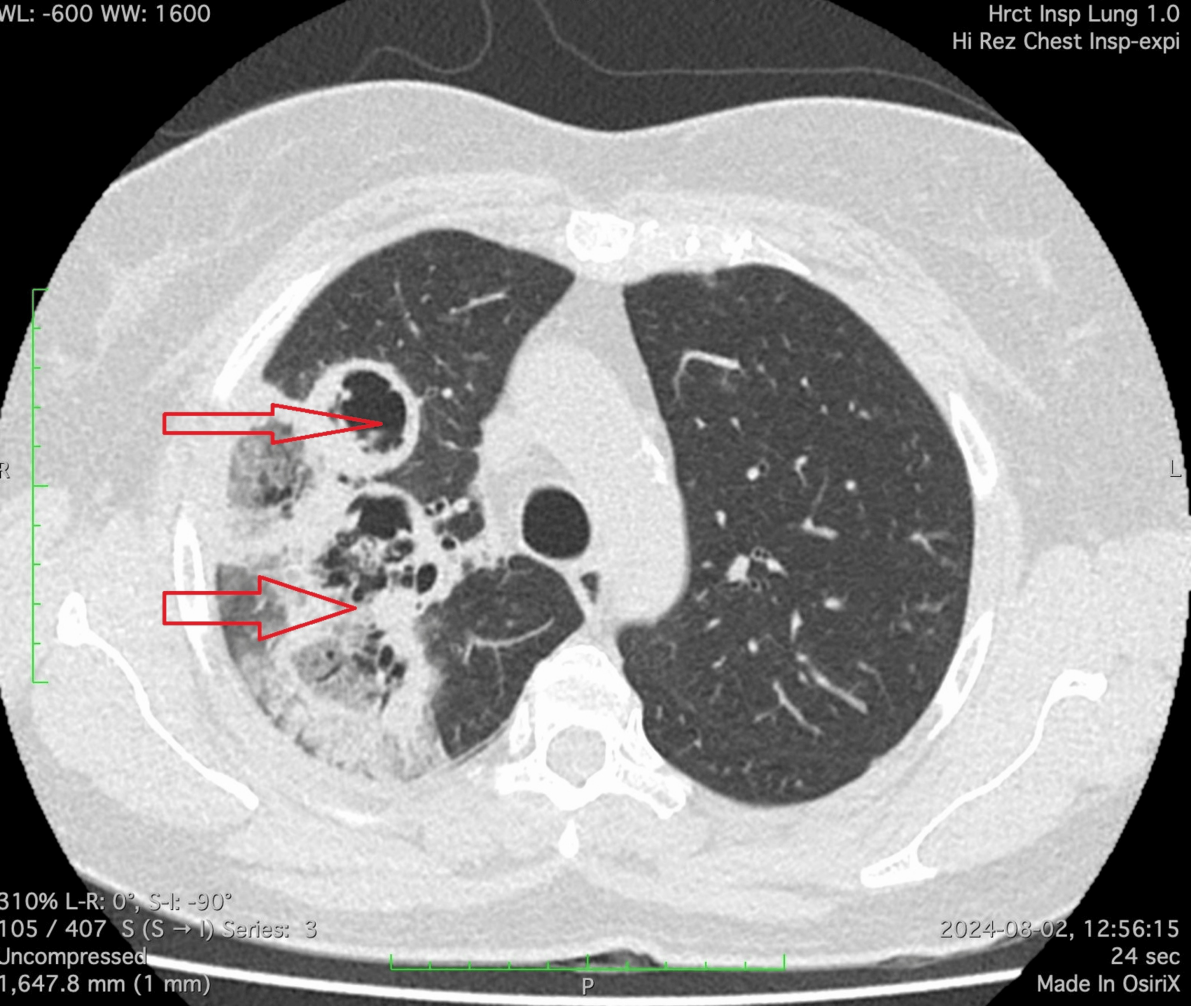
Contrast-enhanced CT showing a thick-walled cavitary lesion in the right upper lobe (red arrow). The internal content appears heterogeneous, with necrotic areas. Surrounding consolidation and mild ground glass opacities can be seen, suggestive of an aggressive infectious process.
